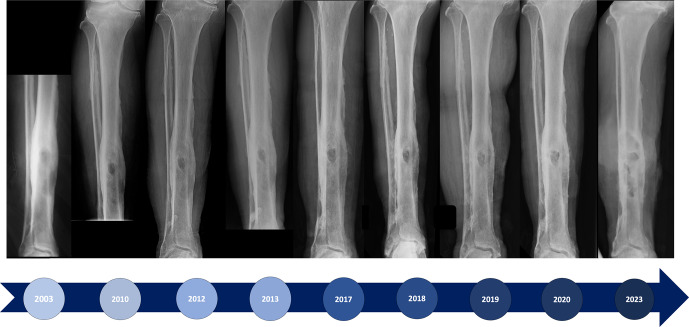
Anterior–posterior radiographs from 2003–2023.
